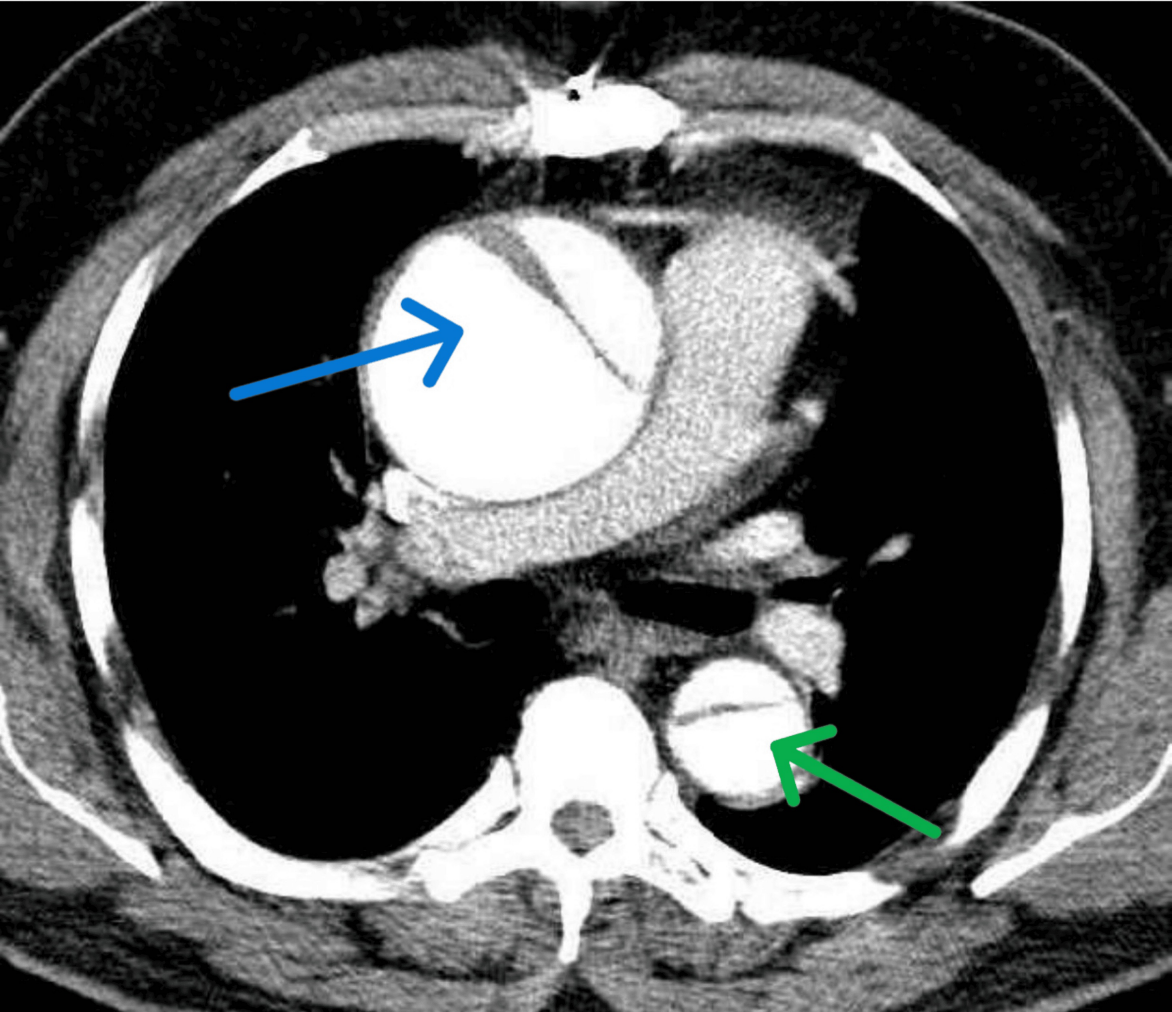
Chest CT (axial view) revealed ascending aorta dissection (blue arrow) and descending thoracic aorta dissection (green arrow).
